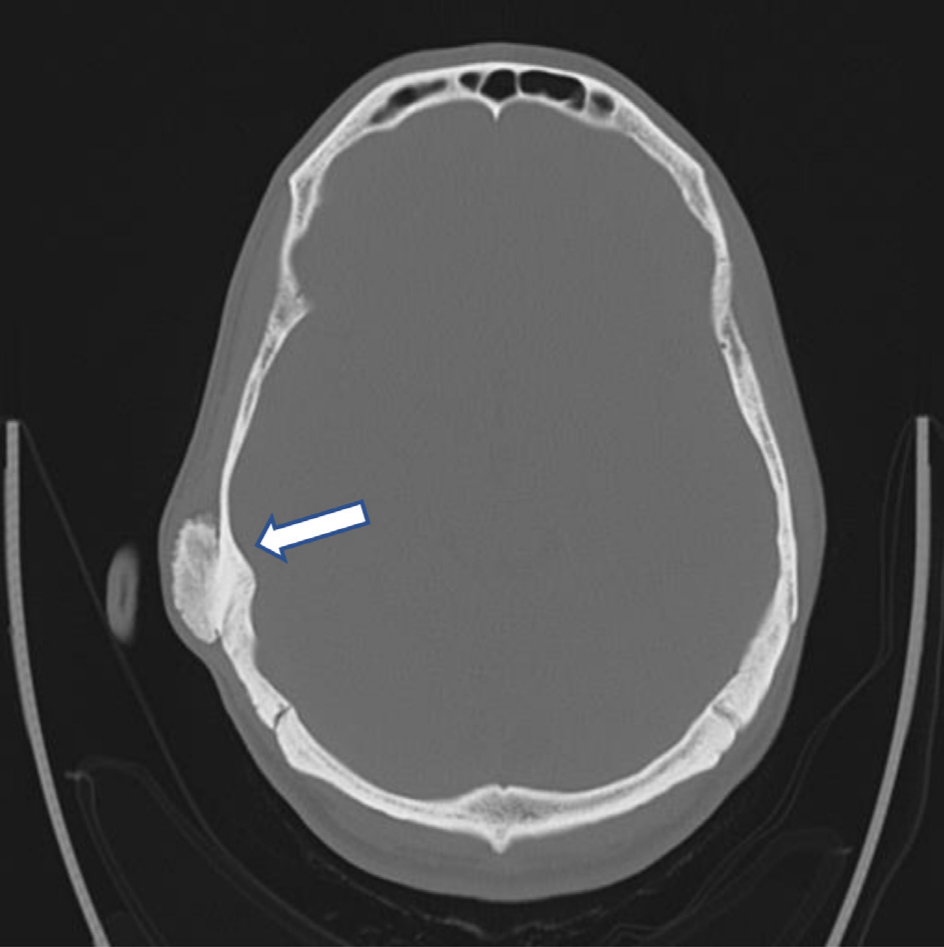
Noncontrast CT shows a benign-appearing hyperostotic lesion measuring 3.0 × 3.0 × 1.1 cm (white arrow) involving the outer table of the right calvarium. There did not appear to be an intracranial component, although some focal bone-like thickening was present in the area. There was no evidence of extracranial soft tissue swelling or mass adjacent to the mastoid bone.
